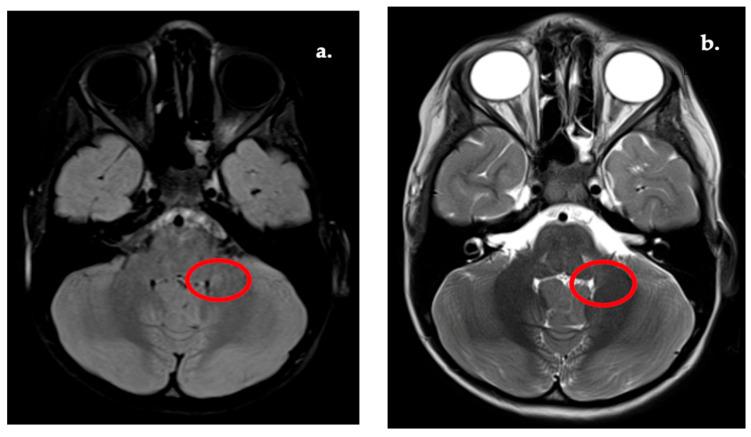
Infratentorial region WMSA—e.g., cerebellum. (a) FLAIR image. (b) T2w image.
